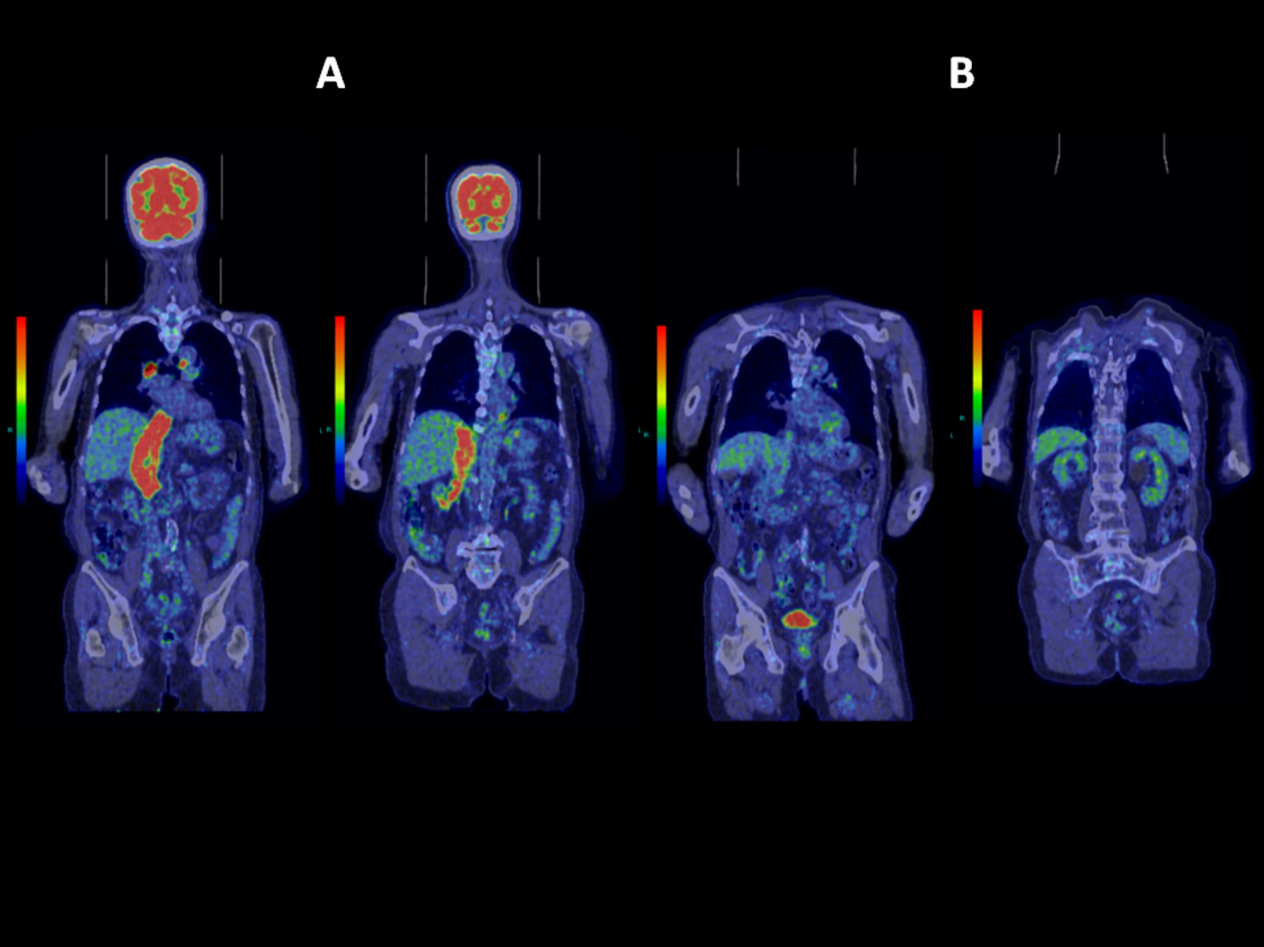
FDG-PET/CT scan before and after treatment. (A) FDG uptake is shown in red spot, revealing IVC tumor, arising from the lower pole of the right kidney, and two lymph node metastases. (B) One year after treatment. FDG uptake is not shown in IVC or kidneys. FDG-PET, fluorodeoxyglucose-positron emission tomography; IVC, inferior vena cava
